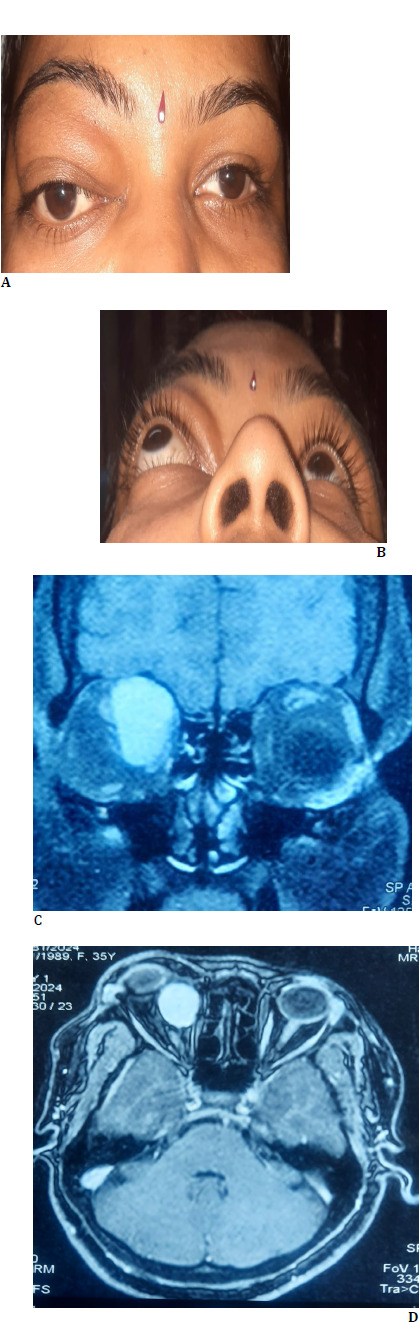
A, B: Clinical photograph demonstrating right eye proptosis in the primary and worm’s view positions. C, D: MRI orbit and brain image showing a well-defined intraconal lesion along the superomedial aspect of the right orbit in the coronal and axial scan
